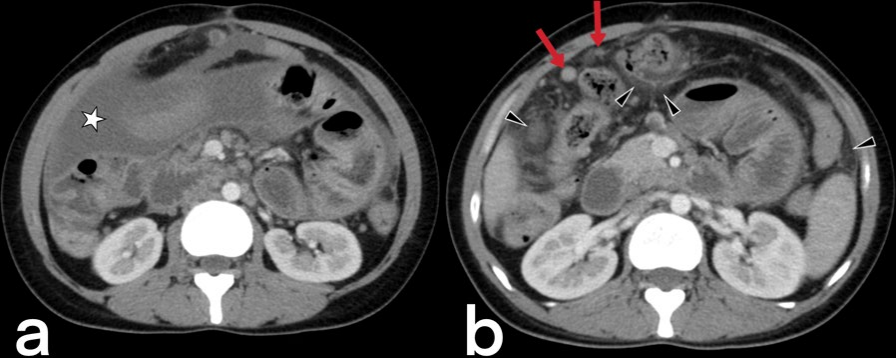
A 33-year-old female. Axial post-contrast CT images (a, b) demonstrate omental nodularity, mesenteric fat stranding (arrowheads), and ascites (star). Intraabdominal lymphadenopathies are also evident (arrows) in image b
